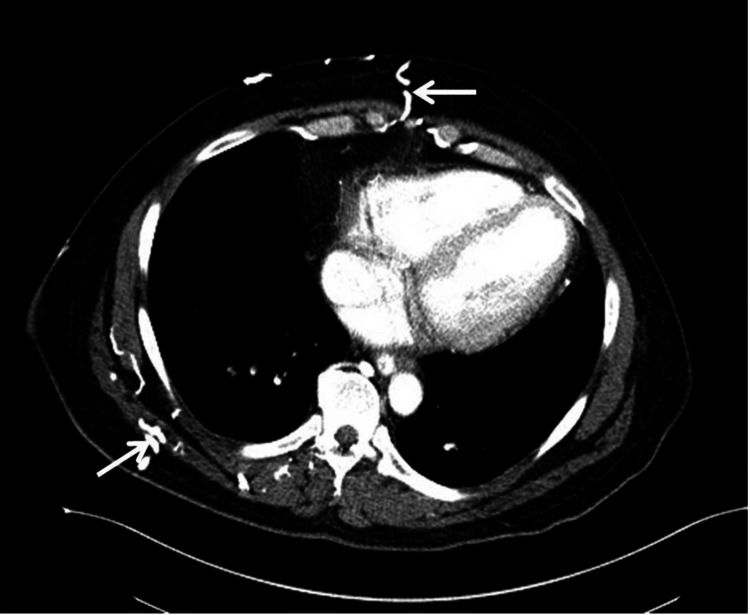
Arterial phase contrast-enhanced CT demonstrating collateral internal mammary vessels along the anterior thoracoabdominal wall, which perfuse the quadrate lobe
